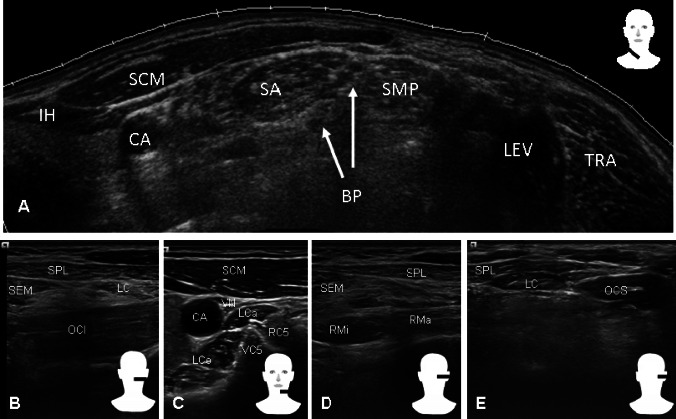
Sonographic appearance of frequently injected muscles (mainly a, b) and more difficult accessible, small, or deeper located muscles (mainly c, d, e). Muscles: *IH* infrahyoid, *SCM* sternocleidomastoideus, *SA* scalenus anterior, *SMP* scalenus medius posterior, *LEV* levator scapulae, *TRA* trapezius, *SEM* semispinalis capitis, *SPL* splenius capitis, *OCI* obliquus capitis inferior, *LC* longissimus capitis, *LCo* longus colli, *LCa* longus capitis, *RMi* rectus capitis posterior minor, *RMa* rectus capitis posterior major, and *OCS* obliquus capitis superior. Others are *CA* carotid artery, *BP* brachial plexus, *VC5* vertebra C5, *RC5* root C5, and *VN* vagus nerve
